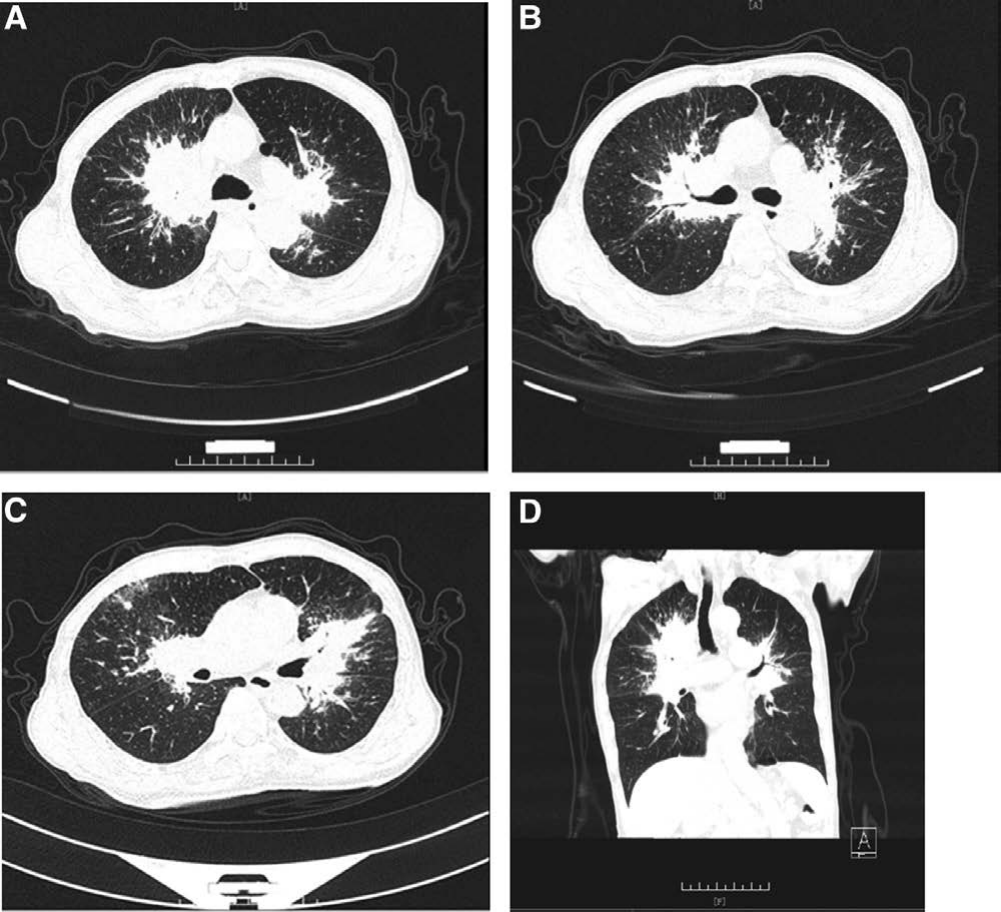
(A–D) 2020-03-19 Chest CT showed multi-locus nodes in the upper right, middle right, upper left and lower left lobes. These shadows were centered on the hilum, with an unclear boundary. Local bronchial lumen stenosis, miliary nodular shadows in both lungs, and an enlarged hilar area were also observed. CT = computed tomography.
